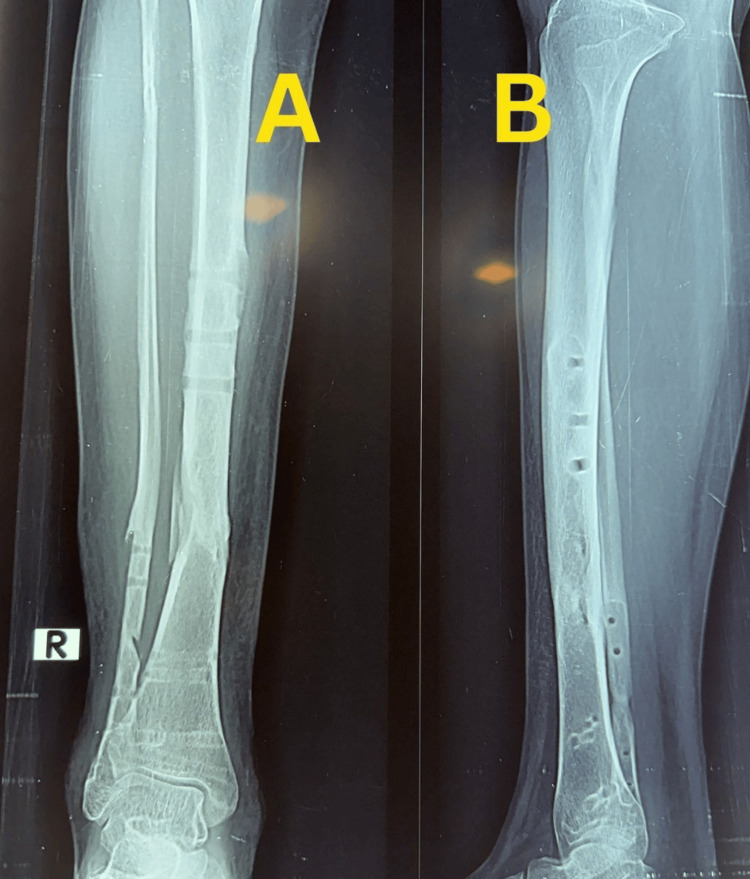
Radiographs after implant removal in Case 3. (A) Anteroposterior view after implant removal showing complete union and remodeling of the distal tibia. (B) Lateral view confirming maintained alignment and consolidated healing following hardware removal.
